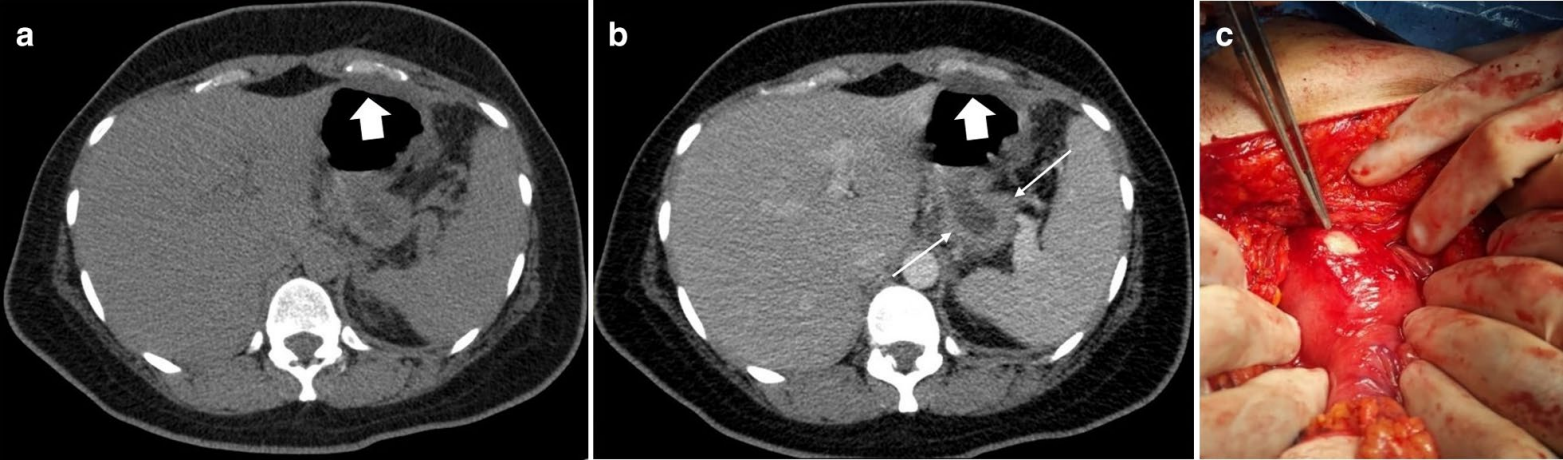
A 42 y/o female with severe epigastric pain 3 days following intragastric balloon insertion for obesity, which was subsequently removed. CT scan was performed 5 days later due to persistent pain and leukocytosis. Pre (a) and post (b) IV contrast CT scan display focal adhesion of gastric body to anterior abdominal wall with mild mural thickening (thick arrows), demonstrating no enhancement on post contrast images compared to the rest of enhancing gastric wall (thin arrows) (grey pattern). c Laparotomy demonstrated anterior gastric wall thinning with pale creamy appearance along with focal perforation due to pressure necrosis from prior balloon placement. The anterior gastric wall was locally resected and repaired
